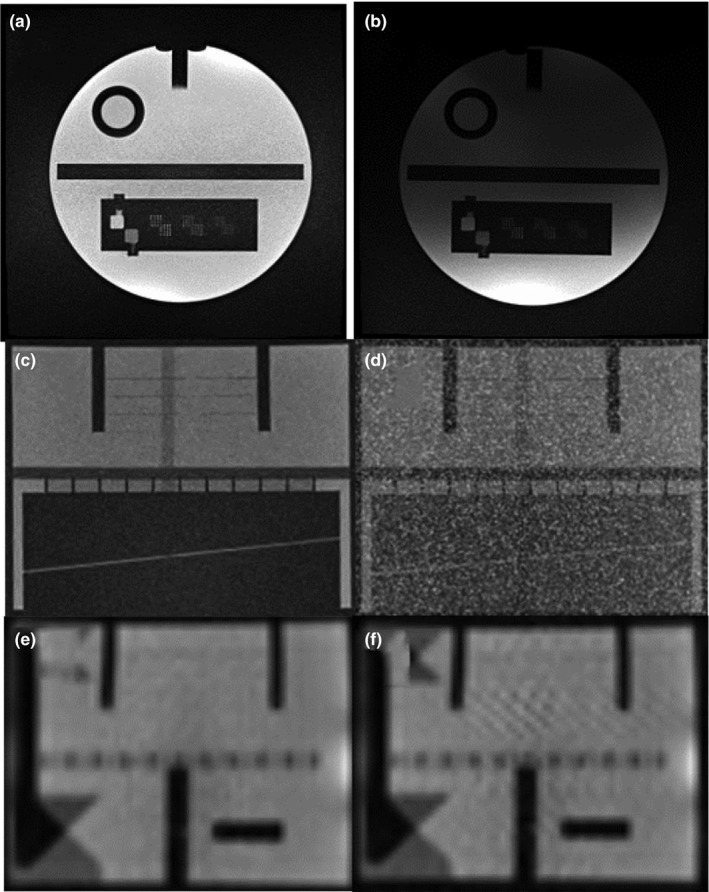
Comparison of MRIs acquired on MRI‐^60^Co with operational (a, c, e) and defective (b, d, f) torso array coils. (a, b) Axial T_1_‐weighted MRIs (TE/TR: 20/500 ms, 90^0^, 1 × 1 × 5 mm, 78 Hz/pixel, 260 s) with (b) defective anterior torso coil with severe signal loss. (c, d) Coronal T_1_‐weighted MRIs (TE/TR: 20/200 ms, 90^0^, 1 × 1 × 5 mm, 78 Hz/pixel, 53 s) with (d) excessive noise. (e, f) 2D sagittal TrueFISP (TE/TR: 1/2 ms, 60^0^, 3.5 × 3.5 × 7 mm, 1335 Hz/pixel, GRAPPA 2, 0.25 s) with (f) herringbone artifact (near center) indicative of RF spike noise. MRI, magnetic resonance imaging.
